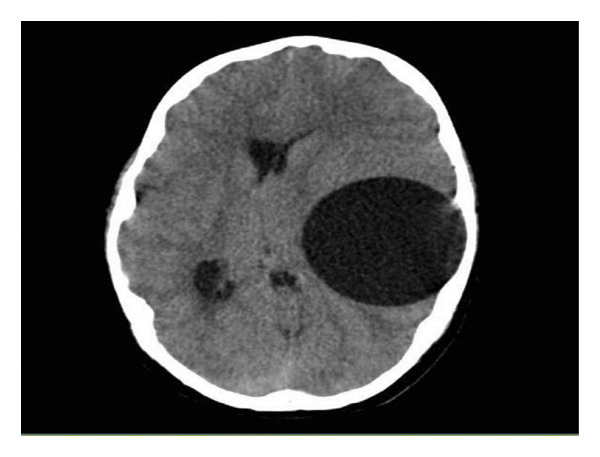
Brain MRI shows a large brain hydatid cyst on the left temporal lobe.
